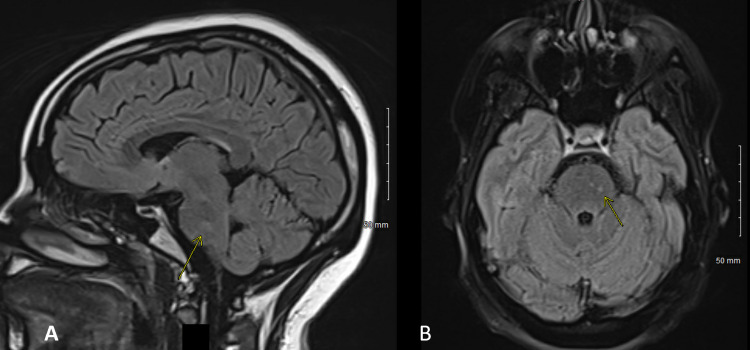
MRI FLAIR images in the sagittal (A) and axial (B) planes demonstrating lesions in the pons (arrows) MRI: magnetic resonance imaging; FLAIR: fluid-attenuated inversion recovery
